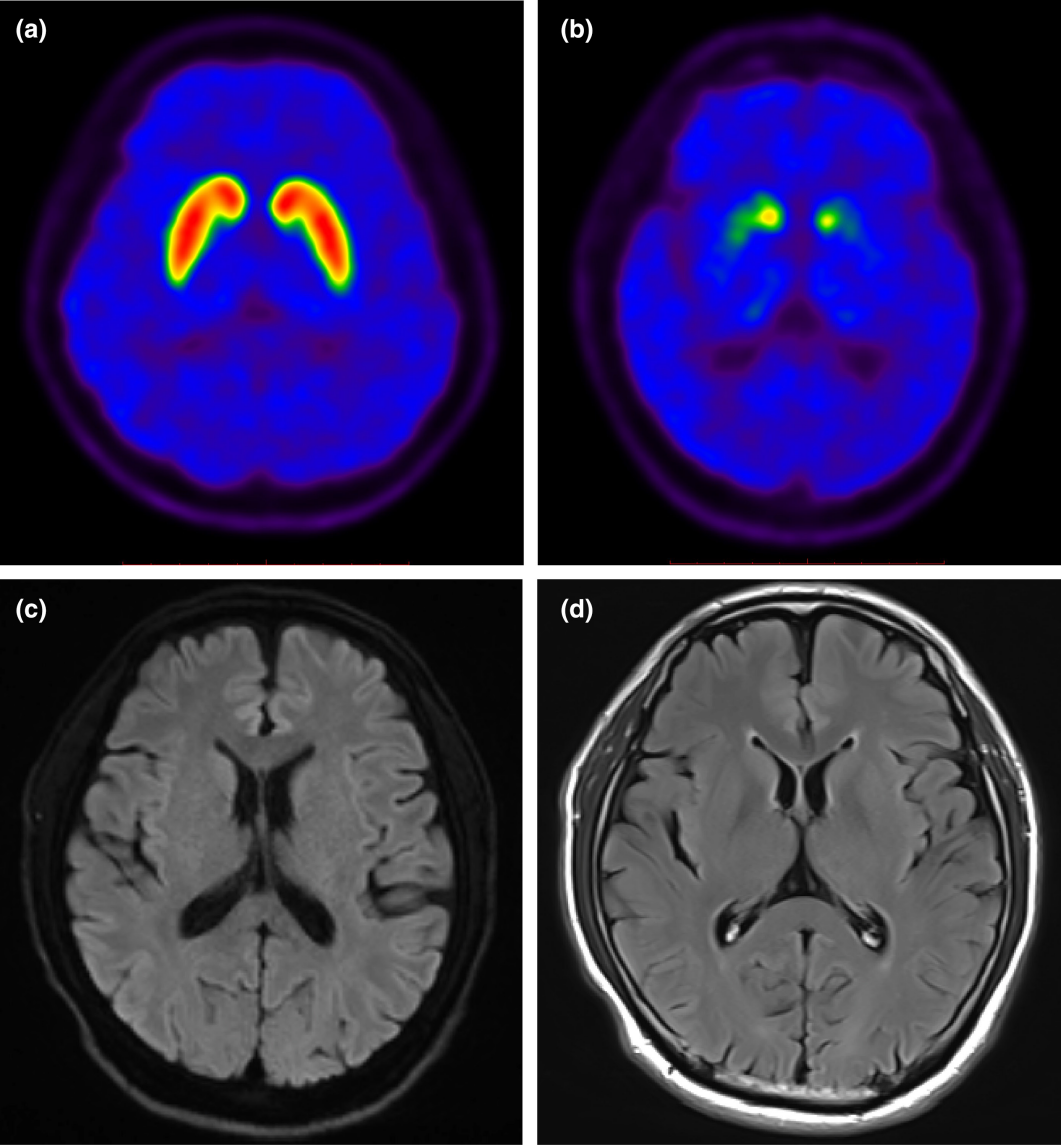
Representative positron emission tomography (PET) and brain magnetic resonance imaging of Parkinson disease harboring *NOTCH2NLC* GGC repeat expansions. Representative axial PET images of ^11^C‐2β‐carbomethoxy‐3β‐(4‐fluorophenyl) tropane (^11^C‐CFT) uptake in a healthy control (a) and PD‐11 (b) show a graded and asymmetrical reduction in dopamine transporter binding (^11^C‐CFT) in the putamen. (c, d) Representative brain diffusion‐weighted imaging images (c) and fluid‐attenuated inversion recovery images (d) of PD‐11, harboring the *NOTCH2NLC* GGC repeat expansions.
